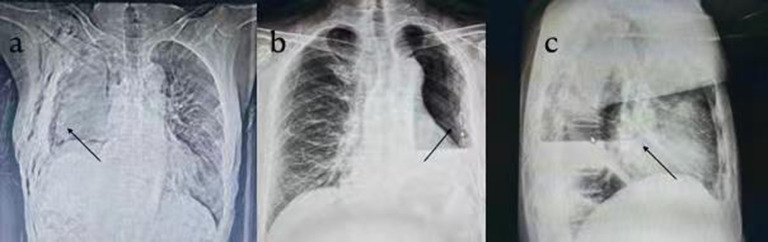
Chest radiography performed on Day 2 after U-VATS-P in patients who received one 8.5-Fr pigtail catheter for postoperative continuous open gravity drainage. The arrow indicates that one 8.5-Fr pigtail catheter was placed in the thoracic cavity. a The patient underwent right U-VATS-P. b c The patient underwent left U-VATS-P
